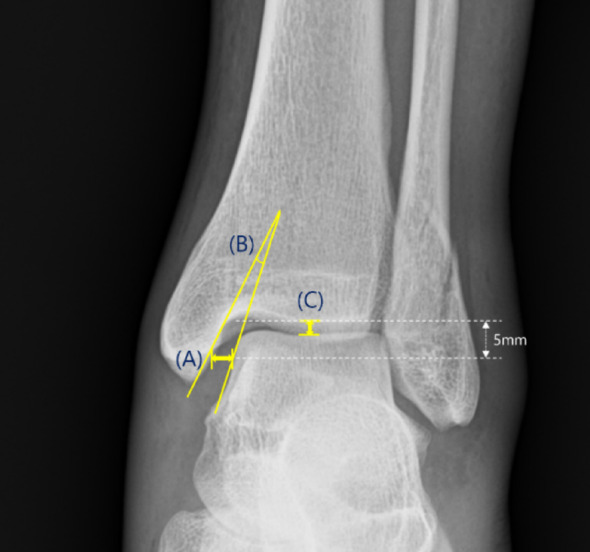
Preoperative radiographic measurements on gravity stress views. A Medial clear space (MCS) is measured as the distance between the medial border of the talus and the lateral border of the medial malleolus, 5 mm below the tibial plafond. B Medial clear space angle (MCA) is defined as the angle formed by the medial articular surface of the talus and the lateral articular surface of the medial malleolus. C Superior clear space (SCS) is measured as the distance between the superior articular surface of the talus and the tibial plafond
